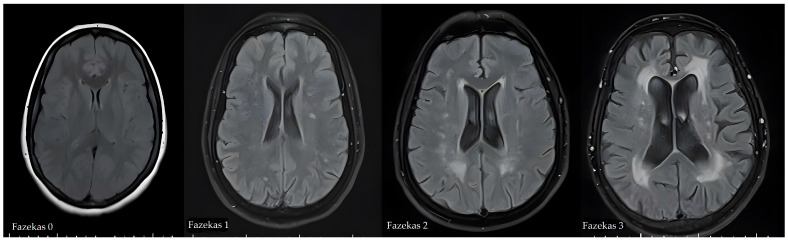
Axial FLAIR images showing the classification of white matter hyperintensities on the four stage Fazekas scale: the healthy brain (Fazekas 0) contrasted with punctiform (Fazekas 1), early confluent (Fazekas 2) and diffuse confluent (Fazekas 3) white matter hyperintensities.
